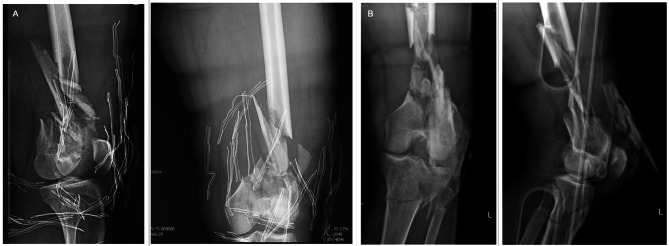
Pre-op X-rays. (A) Patient One (B) Patient Two
